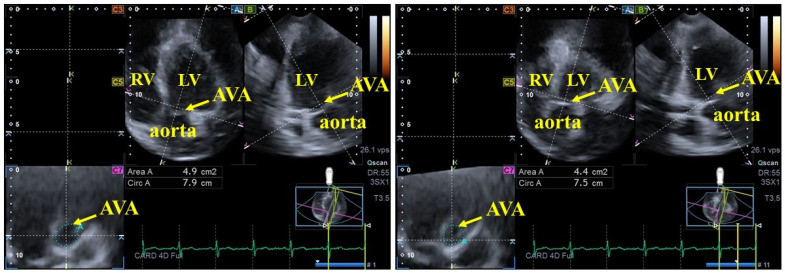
Evaluation of end-diastolic and end-systolic aortic valve annular dimensions and aortic valve annular spatial displacement, represented by aortic valve annular plane systolic excursion, via three-dimensional speckle-tracking echocardiography. Abbreviations: AVA, aortic valve annulus; Area A, AVA are; Circ A, AVA perimeter; LA, left atrium; LV, left ventricle; RV, right ventricle.
